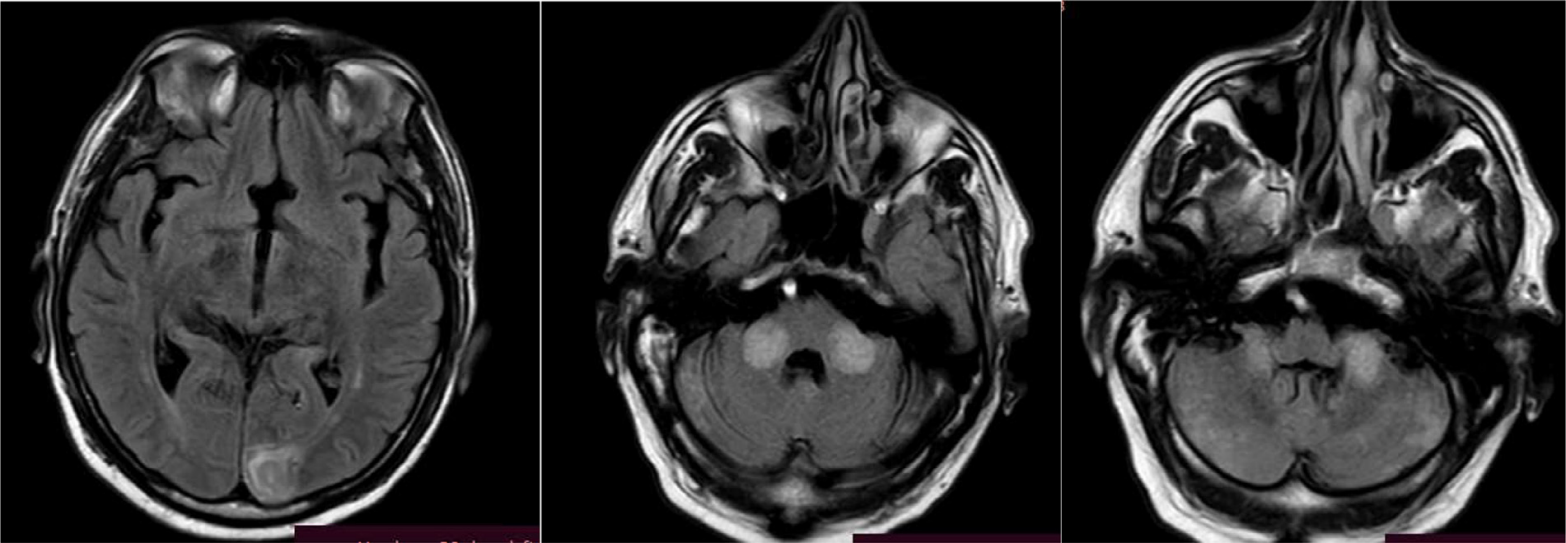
Presents an axial FLAIR sequence MRI of the cranial cavity, highlighting hyperintensities observed in the left occipital lobe.Figs. 2 and 3 Depict bilateral multifocal signal anomalies localized to the infratentorial region, as visualized through the FLAIR sequence.
